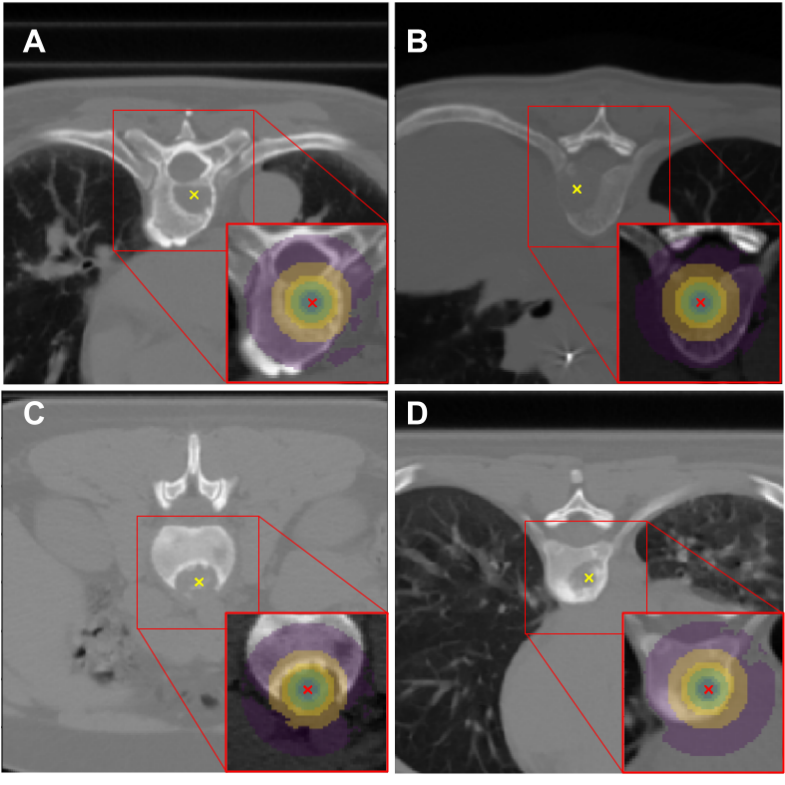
Examples of segmented nested spherical regions of interest (ROIs) with the Hounsfield units threshold applied on computed tomography images of patients with painful (A, B) and painless (C, D) bone metastases lesions. Nested ROIs with diameters of 50, 30, 20, 15, 10, and 7 mm are shown in the insets as different hues.
